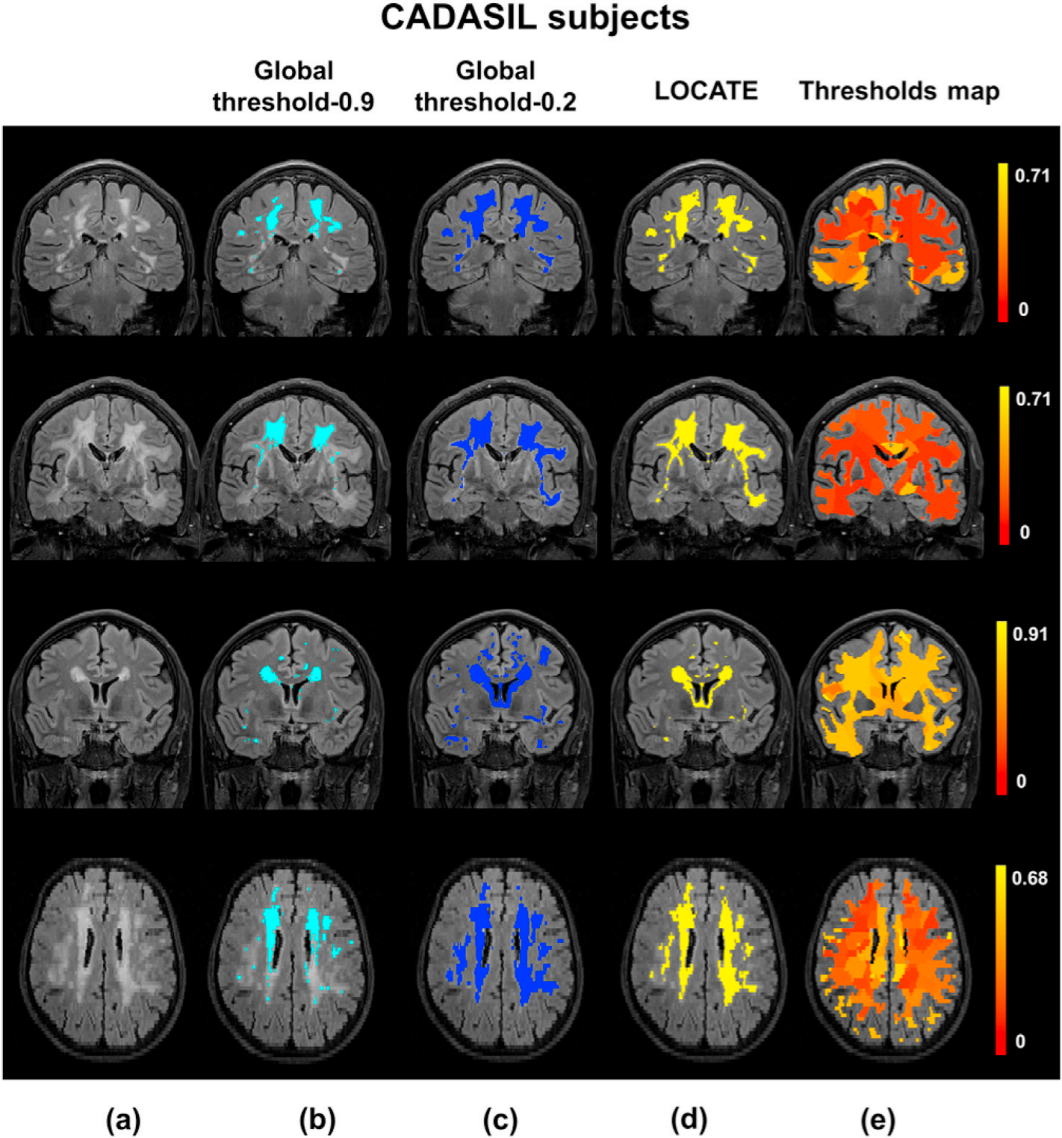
Example results of LOCATE on the CADASIL data. (a) FLAIR image, (b) global thresholding at 0.9 (light blue), (c) global thresholding at 0.2 (dark blue), (d) LOCATE results (yellow) and (e) threshold maps obtained from LOCATE (red-yellow). Note that the threshold maps shows the heterogeneity in the lesions probabilities in various regions of the white matter.
